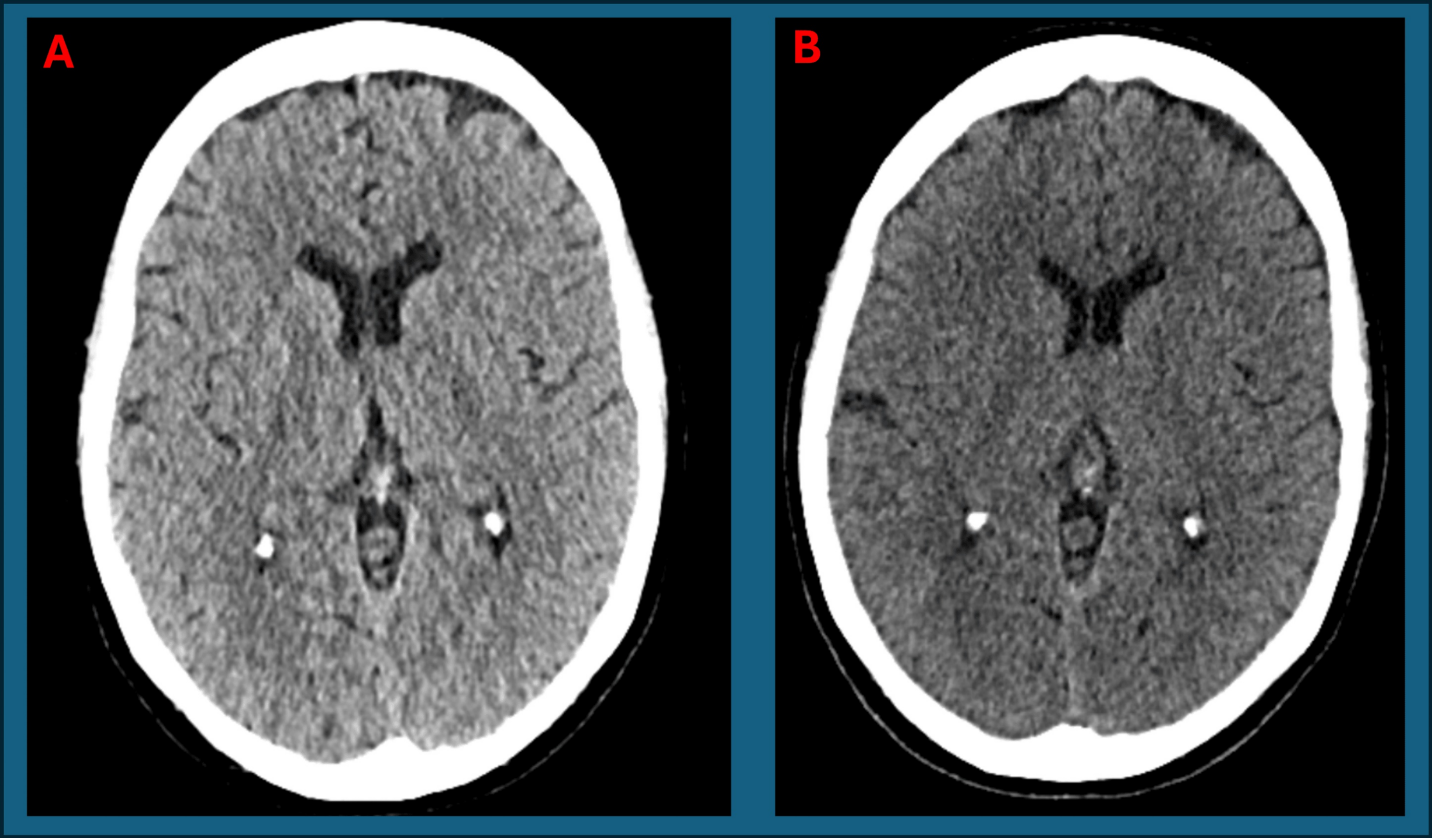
A: Normal axial CT brain from first presentation. B: Normal axial CT brain from second presentation
